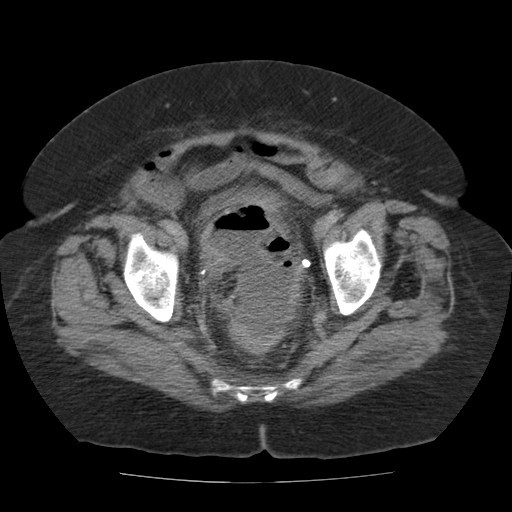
Computed tomography scan of the abdomen and pelvis (axial section) demonstrating the diverticular abscess extending anterior to rectum.
